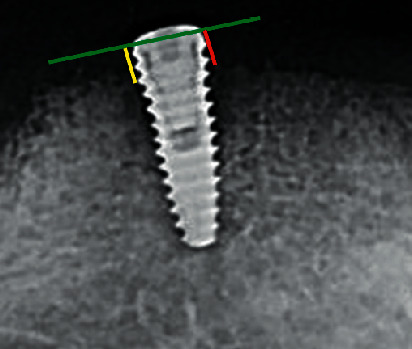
Radiographic tracing for periapical X-ray film. The mesial and the distal lines (red and yellow, respectively) parallel for each other and perpendicular to reference (green) line.
